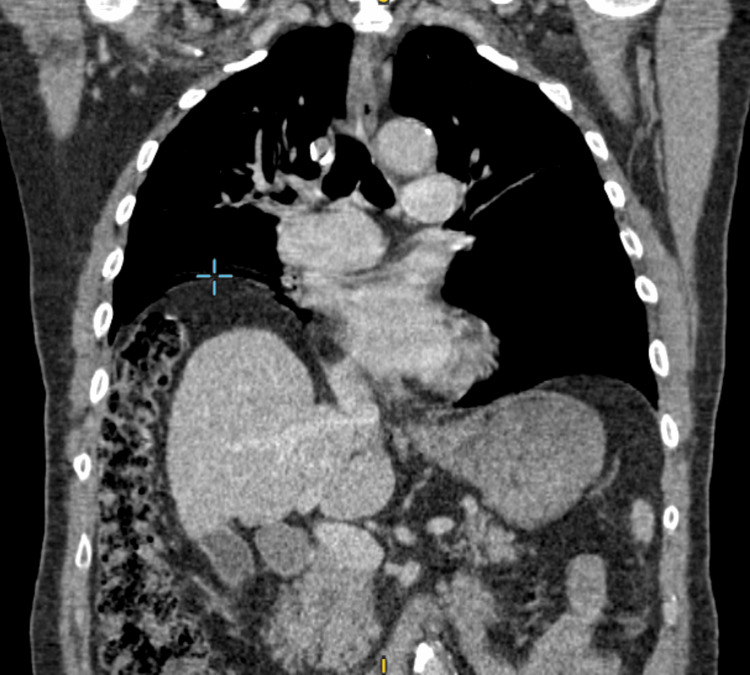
CT thorax coronal view in 2025, showing elevated right hemidiaphragm at the highest point (blue crosshair) with large bowel characterized by typical colonic haustral markings herniating between the liver and the right hemidiaphragm. CT, computed tomography.
